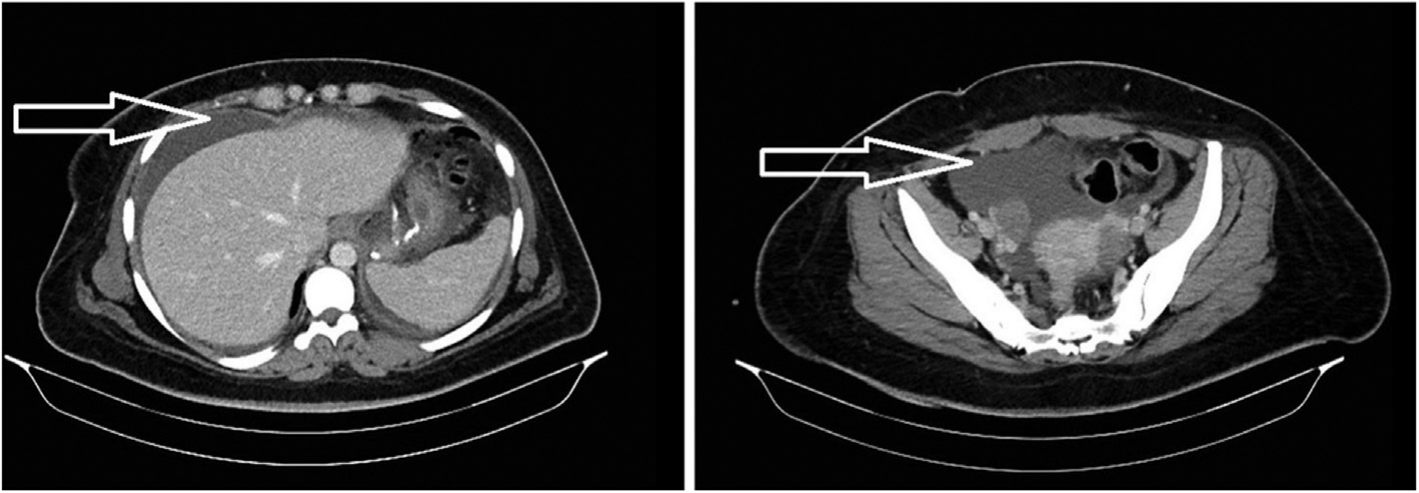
CT scan of abdomen showing fluid in the subhepatic and Douglas spaces (white arrow).
